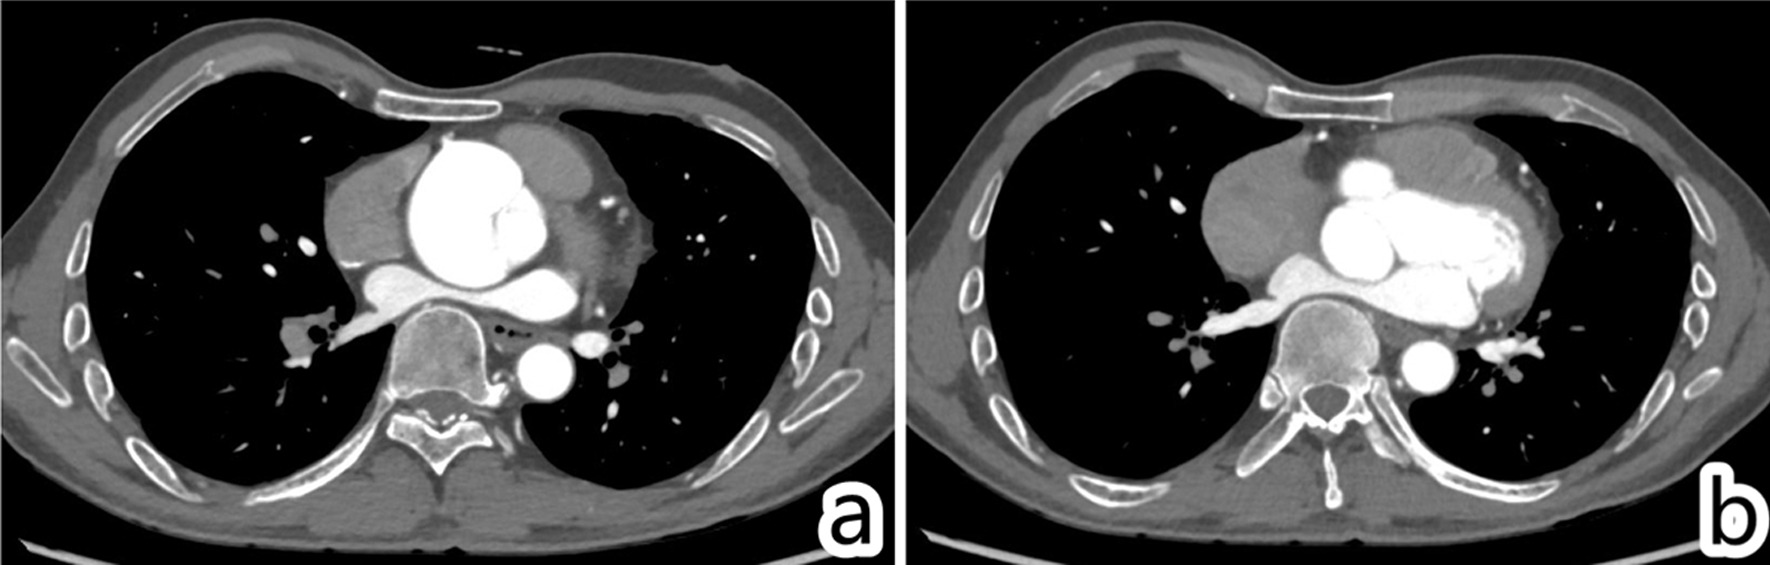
Chest computed tomography. a Aortic sinus aneurysm, approximately 54 mm in diameter; b the LA was compressed by the thoracic spine and aortic sinus aneurysm, with an anteroposterior diameter of only 20 mm
